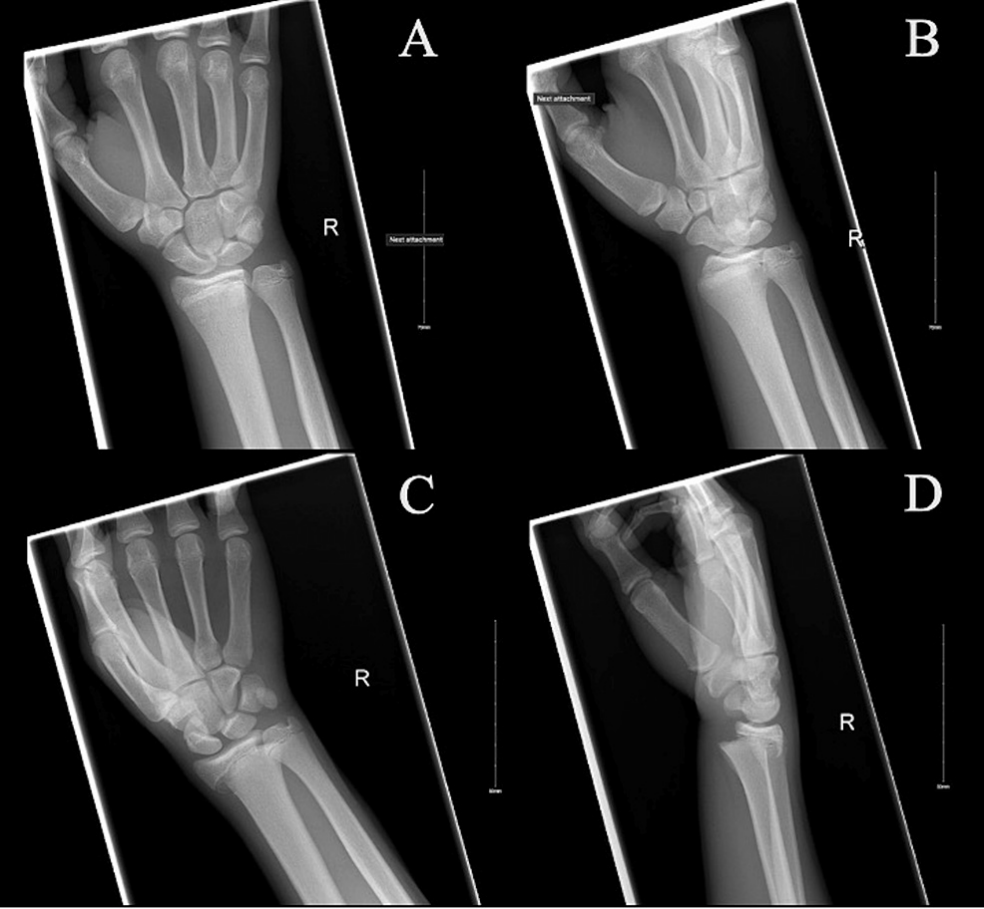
Right wrist radiographs pre-reduction Postero-anterior (A), oblique (B and C), and lateral (D) radiographs of right wrist pre-reduction demonstrating a right Salter-Harris II distal radius fracture with dorsal angulation.
